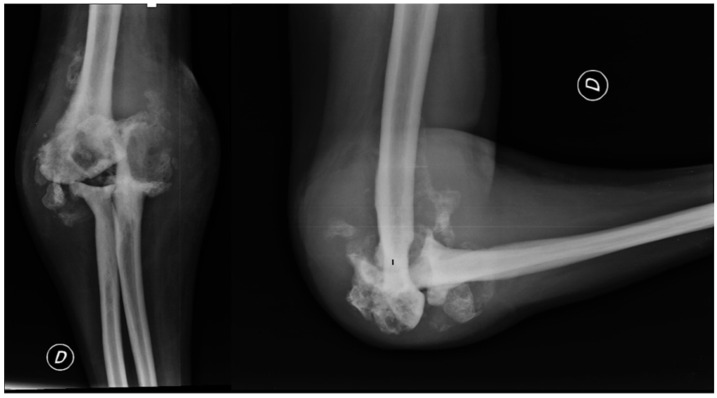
X-ray anteroposterior and lateral views of the right elbow. First radiological presentation with subversion of the local anatomy of the bones and soft tissue around the elbow joint.
